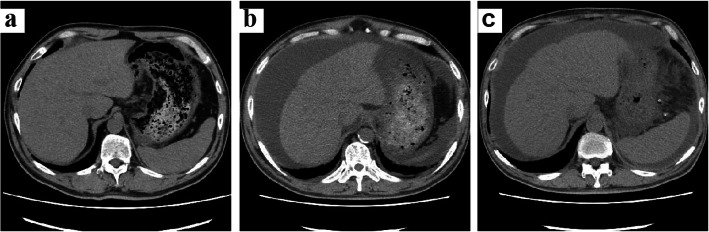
Abdominal computed tomography (CT). a (Day 0): the liver is enlarged. The biliary system obstruction mechanism is not recognized. The liver volume measured by CT is 1973 cm^3^. b (Day 37): the liver atrophied with massive ascites. The liver volume is 1419 cm^3^. c (Day 284): the liver is regenerating and growing. Ascites are reduced. The liver volume is 1587 cm^3^. The number of days from the date of hospitalization to the date of examination
